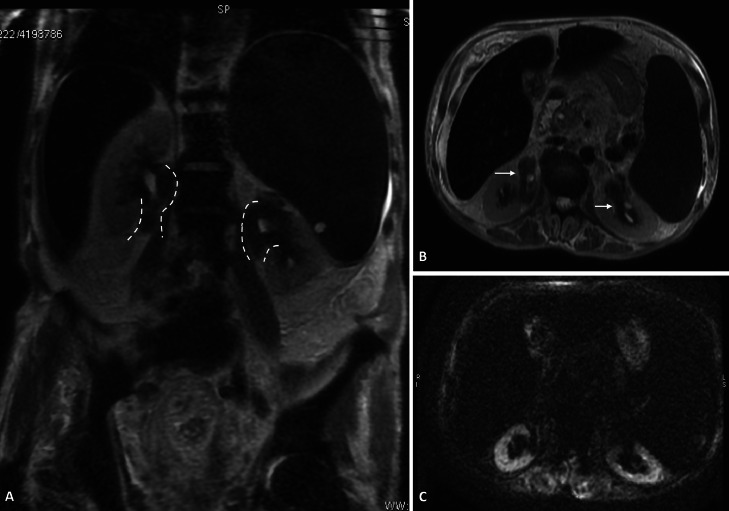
Radiologic follow-up in the same patient of Figure 1. The MR examination, performed 6 months later, confirmed the radiological pattern of bilateral renal lesions along the profile of the ureteropelvic junction (white dotted curved lines), showing low signal intensity on coronal (A) and axial (B) T2-weighted turbo spin-echo images (white arrows). (C) On axial DW image (b = 1000 s/mm^2^) the lesions do not exhibit restricted diffusion.
